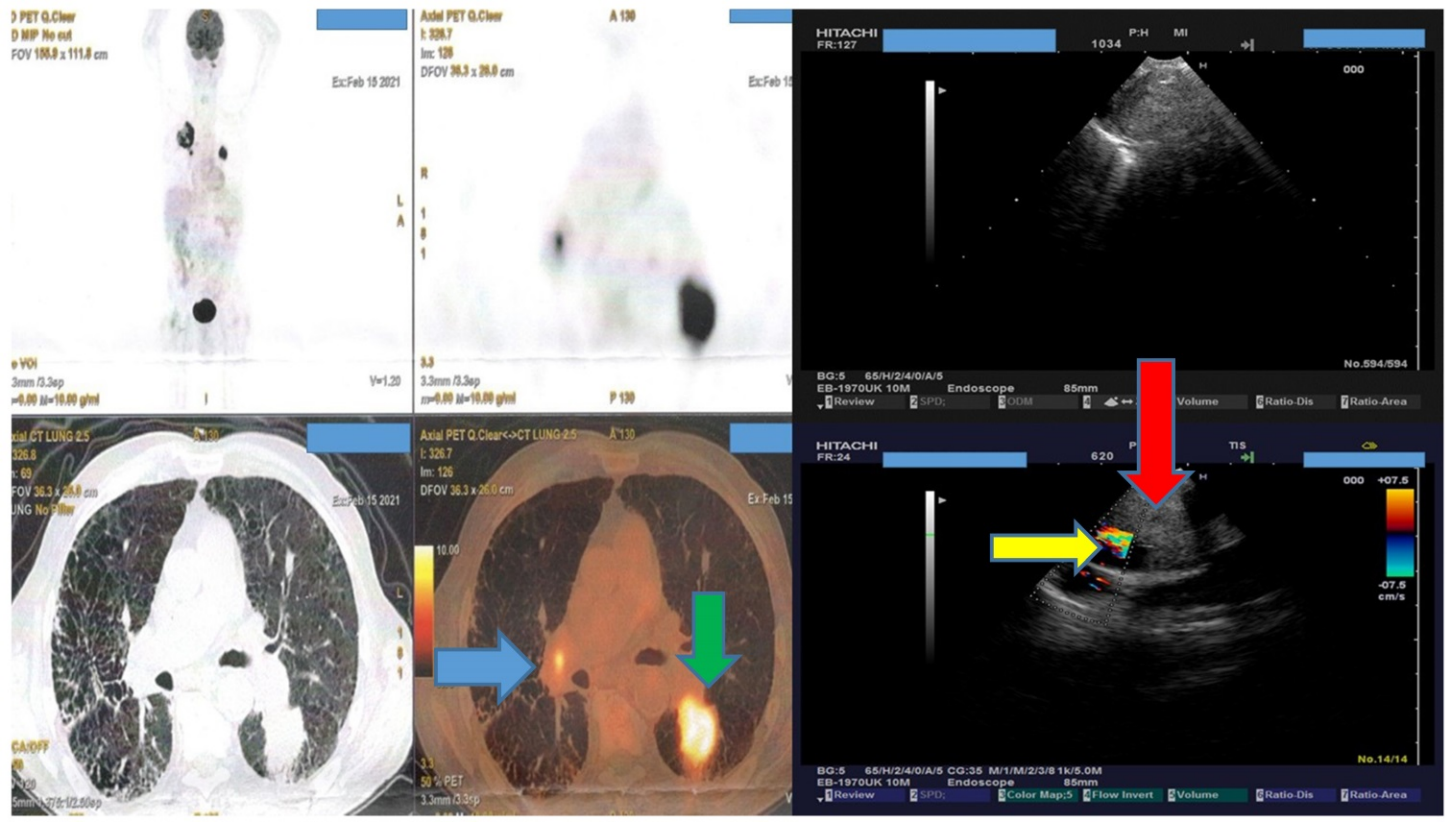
Lymphnode 11L does not have any SUV uptake. Blue arrow indicates a positive lymphnode station L10R, green arrow indicates a lung cancer mass, again red arrow indicates the same lymphnode station on the left (L10R with blue arrow) and red arrow indicates a right pulmonary artery branch next to lymphnode station L10R.
